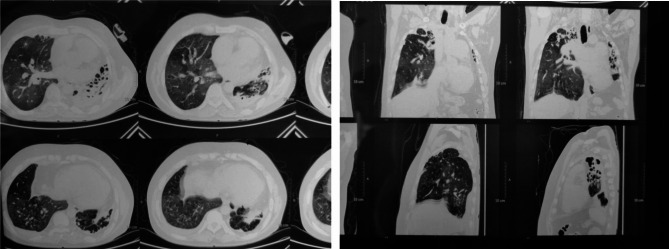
HRCT of chest showing patchy ground glass opacities, cystic bronchiectatic changes indicating post infective sequelae
